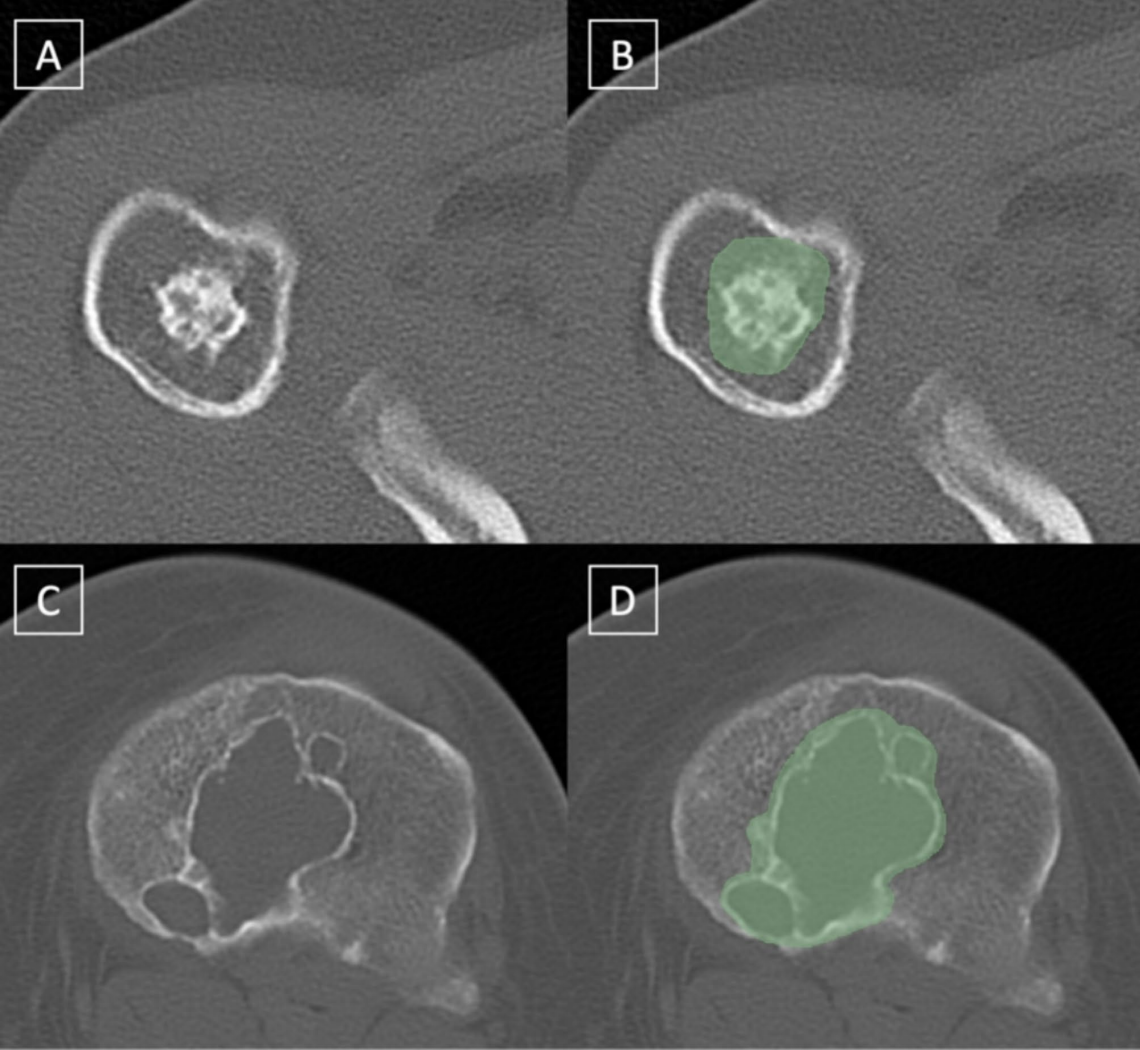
Axial CT Images of patients with an enchondroma (A, B) and a high-grade chondrosarcoma (G2) (C, D) with (B, D) and without the respective segmentations (A, C)
